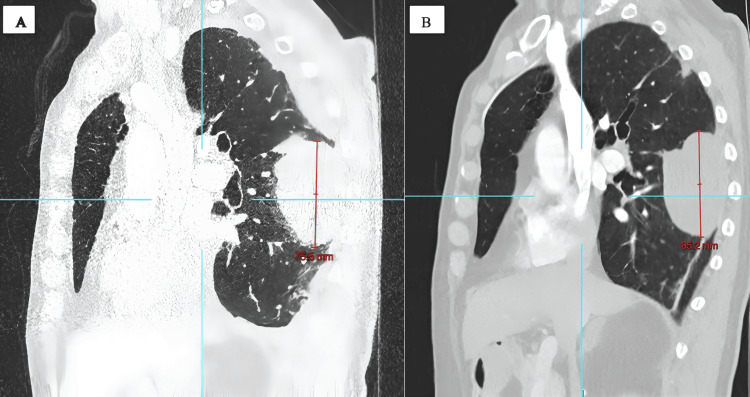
Sagittal CT chest image showing the size of the mass (A) before and (B) after neoadjuvant therapy.
